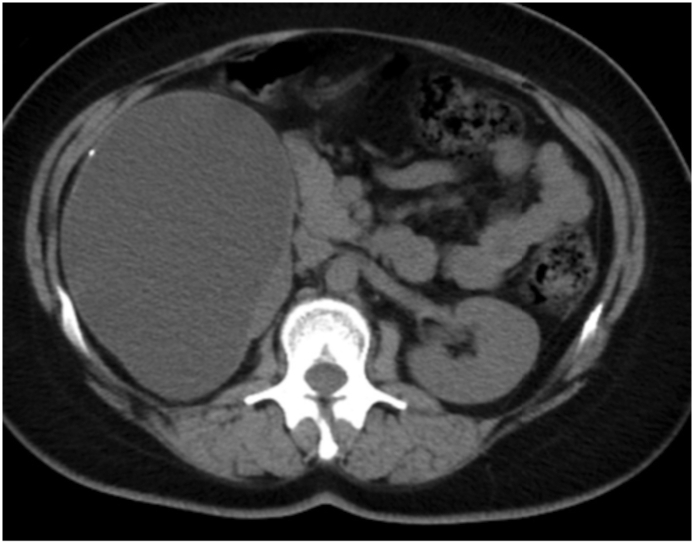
CT showed the recurrence of the renal cyst, measuring 148 mm in diameter.
